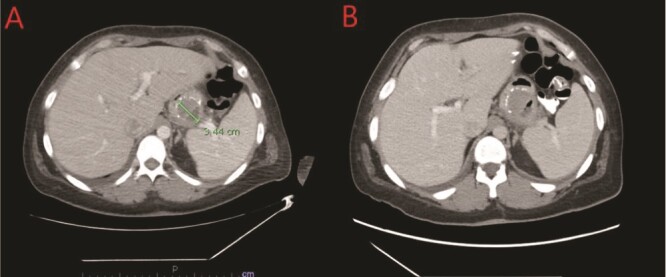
(A) CT showing a complex fluid collection at the proximal greater curvature of the stomach consistent with intramural abscess. (B) Interval improvement in complex fluid collection after endoscopic stent removal.
